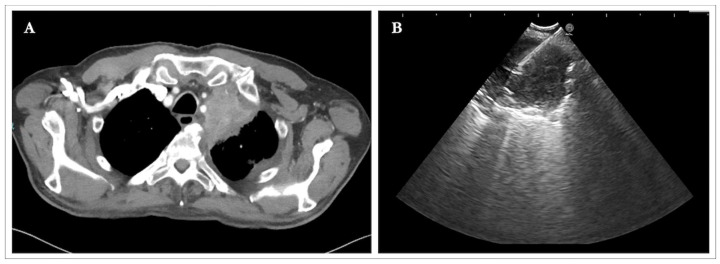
Radiological–endosonographic correlation of a parenchymal lesion sampled by EUS-B-FNA. (A) Chest CT scan showing a lung lesion in the left upper lobe. (B) Endosonographic image of a EUS-B-FNA of the malignant lesion.
